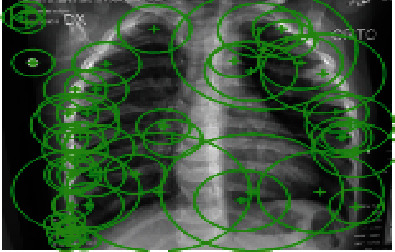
The discriminative SURF features extracted from the X-ray image.
